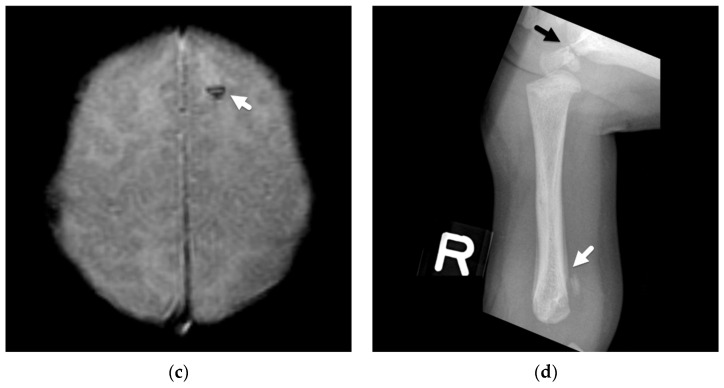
Focal laceration or contusional tear in a 2-month-old former preterm male infant presenting with seizure. (a) Initial axial noncontrast CT demonstrates a small, focal, left frontal lobe laceration/tear with layering posterior hemorrhage (white arrow); (b) axial T1-weighted image shows a hypointense, well defined laceration/tear with subtle layering hemorrhage posteriorly (white arrow); (c) axial multiplanar gradient recalled acquisition in the steady-state (MPGR) image shows the layering posterior hemorrhage (white arrow); (d) follow-up skeletal survey demonstrates healing right acromion fracture (black arrow) and distal humeral periosteal reaction (white arrow) suggesting a healing fracture.
